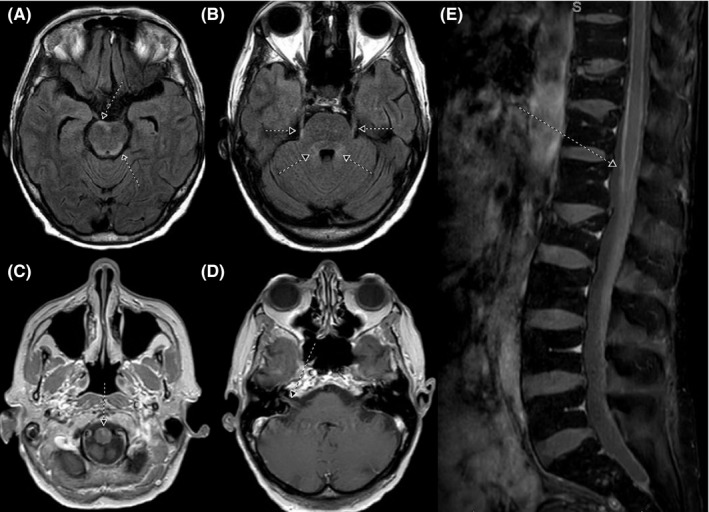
Axial FLAIR sequence shows mild FLAIR signal hyperintensity within the ventral aspect of pons, dorsal brainstem, along the trigeminal nerves, and surrounding the fourth ventricle (arrows, A and B). Axial postcontrast T1 sequence shows leptomeningeal enhancement around the upper cervical cord and enhancement along the right 7th and 8th nerves (arrows, C and D). Postcontrast sagittal fat suppression sequence shows mild leptomeningeal enhancement around the conus medullaris (arrow, E) with an incidental old compression deformity of the L2 vertebral body
